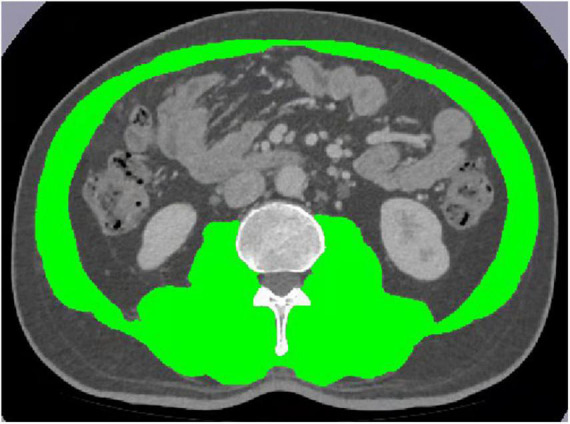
Skeletal muscle mass analysis of computed tomography images on an L3 section by SliceOmatic.
